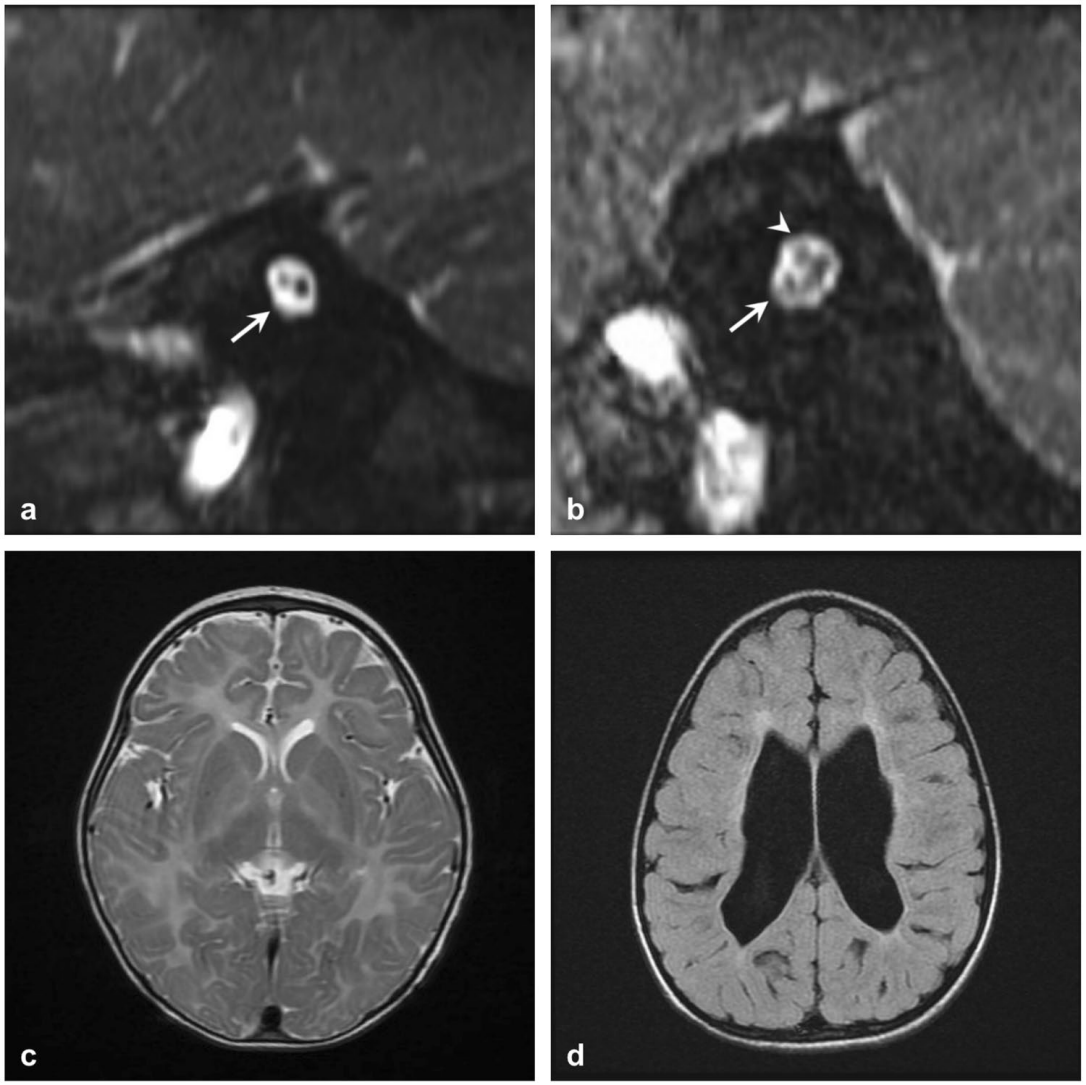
Representative imaging findings on posterior fossa magnetic resonance imaging T2-weighted images. (a) Sagittal view showing cochlear nerve aplasia. The arrow indicates the absence of the cochlear nerve. (b) Sagittal view showing cochlear nerve hypoplasia. The cochlear nerve (arrow) is thinner than the facial nerve (arrow head). (c) Axial view showing hypomyelination of the frontoparietal central parenchyma. (d) Axial view showing diffuse parenchymal loss.
